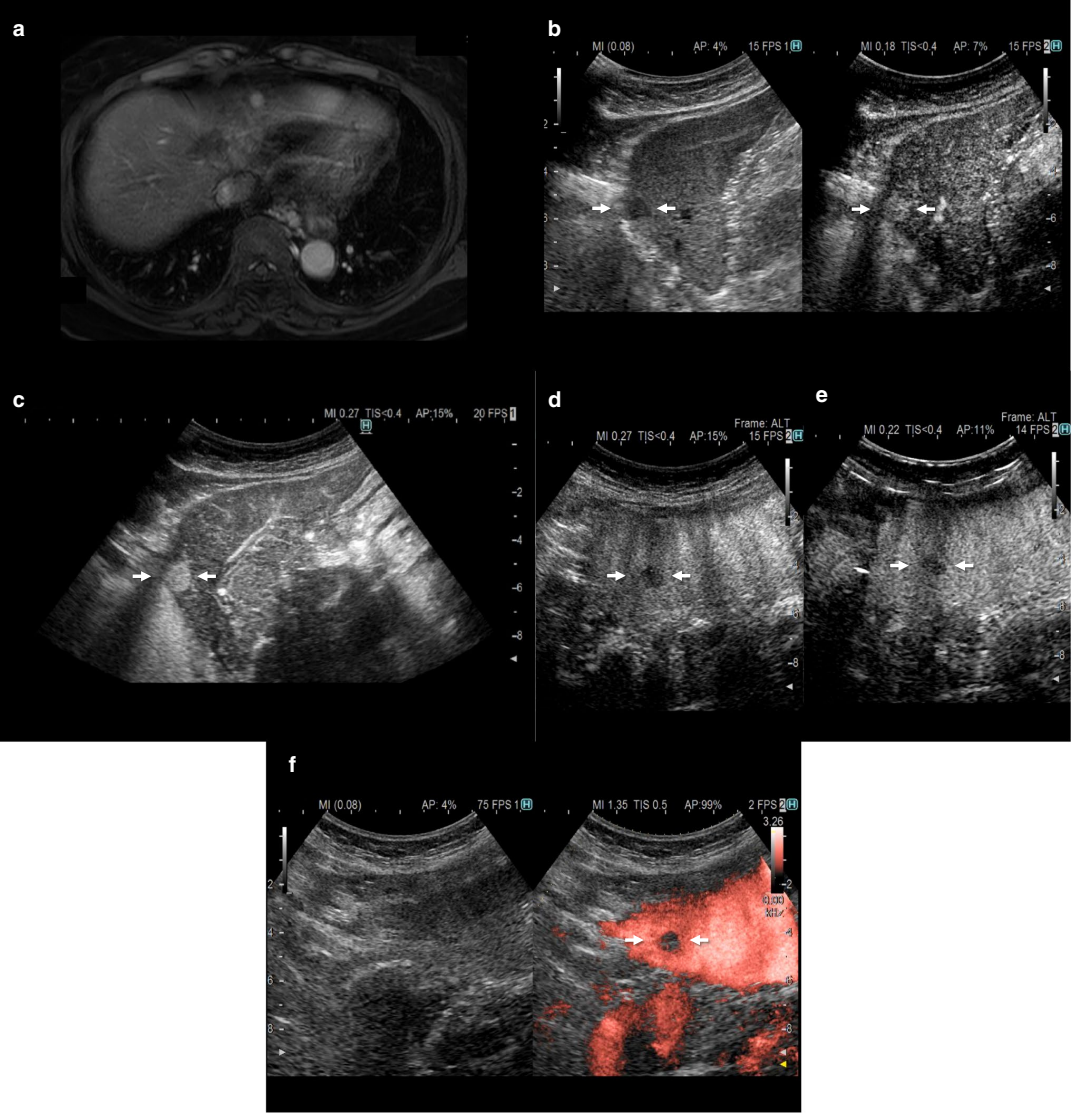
A case of newly developed HCC (maximum diameter 11 mm) in Segment 2. Hepatobiliary phase contrast-enhanced MRI with gadolinium ethoxybenzyl diethylenetriamine pentaacetic acid (Gd-EOB-DTPA) in the arterial phase shows a high-intensity area (a). This tumor is clearly enhanced with contrast-enhanced ultrasound with Sonazoid in the arterial phase using the phase inversion method (b). However, it is more clearly depicted by low mechanical index (MI) contrast-enhanced tissue harmonic imaging (THI) because of its high spatial and time resolutions (c). In the post-vascular (Kupffer) phase, the tumor is in the hypoechoic area by amplitude modulation, which is the mode most sensitive to contrast-enhanced ultrasound (d). We could also delineate the tumor with the same sensitivity by low MI contrast-enhanced THI (e). In addition, high-MI intermittent Doppler imaging could most clearly depict the tumor in the post-vascular (Kupffer phase) (f). The arrows point to the location of the identified hypoechoic tumor on imaging
